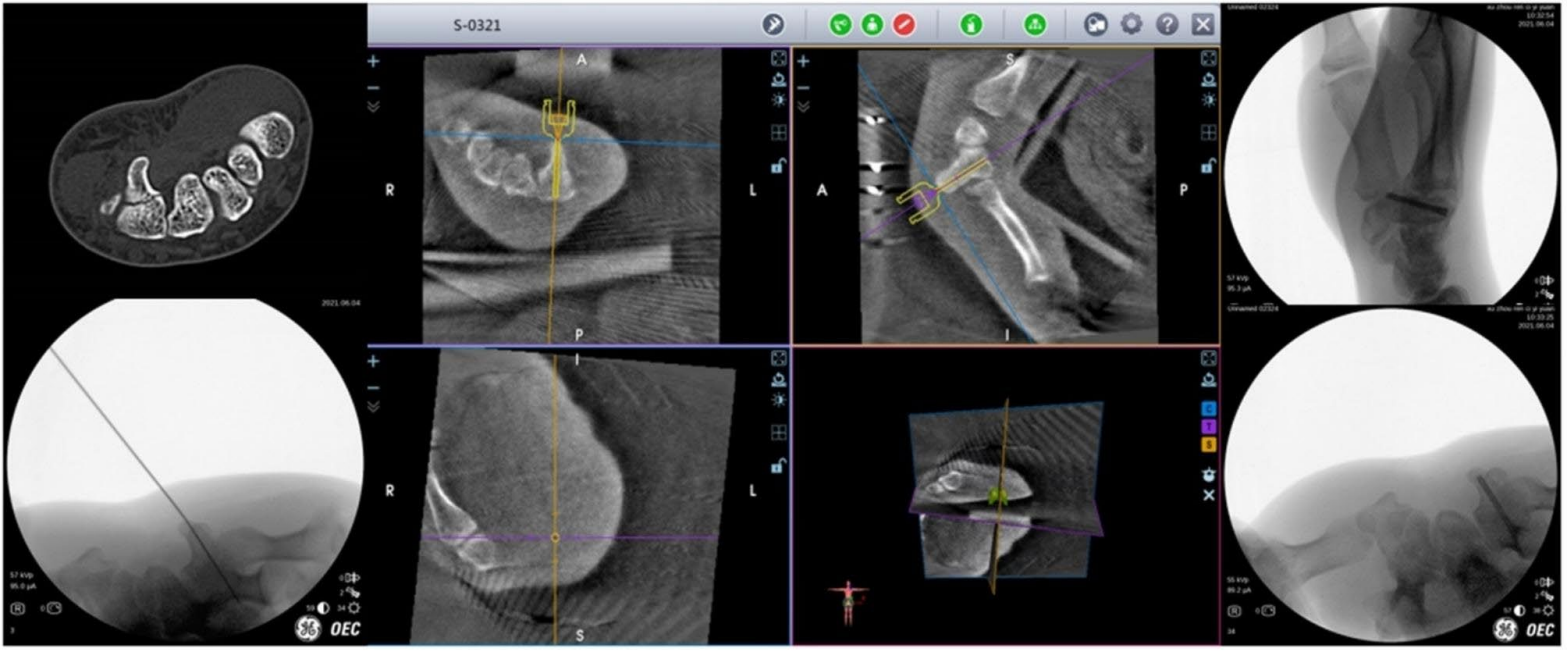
The preoperative CT images of the patient showed a hook of hamate fracture. Guidewire placement is achieved by using TiRobot navigation, and fracture reduction is achieved by using fluoroscopy. A headless cannulated compression screw is then used to rigidly secure the hook of the hamate fracture through a dorsal approach
